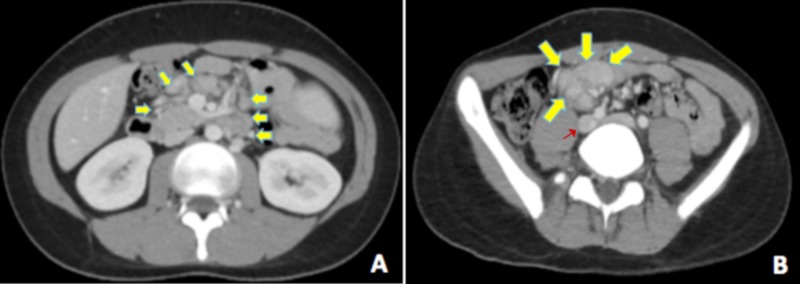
Computed tomography (CT) scan of the abdomen A CT scan in the axial plane showed multiple, enlarged mesenteric adenopathies in a pattern of rounded nodular enhancing masses (yellow arrows) seen at the mesenteric root (4A) and along the right iliac vessels (4B, red arrow).
